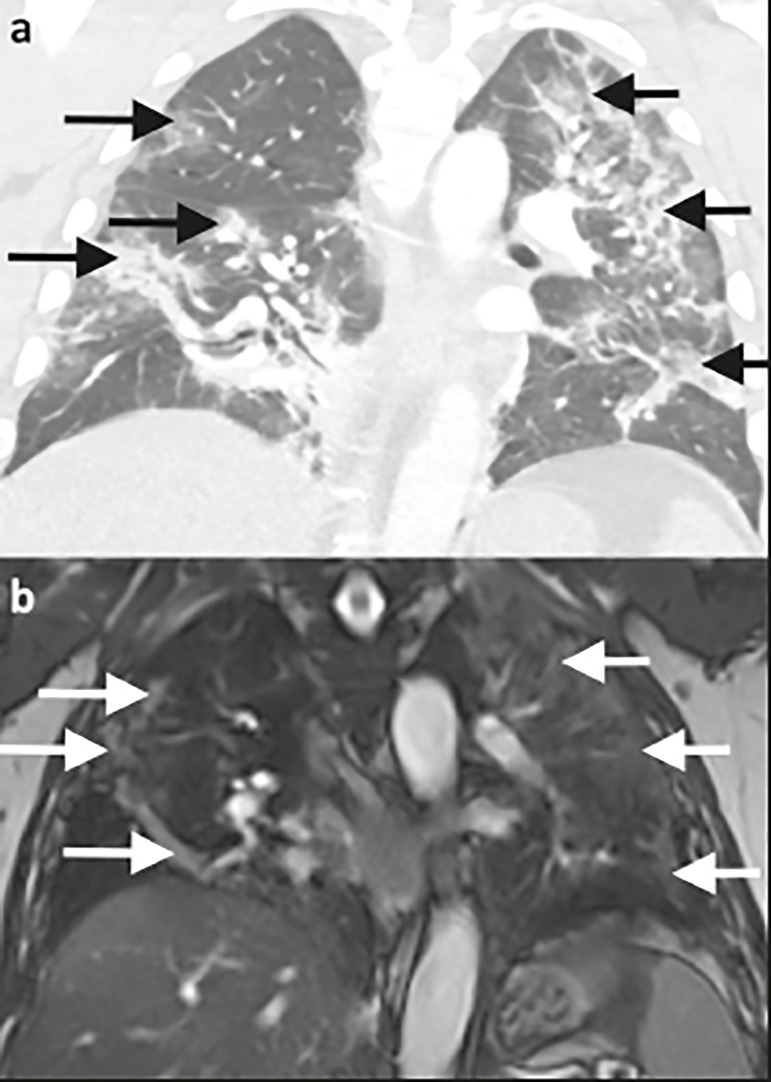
Ability of 1.5 T TrueFISP MRI to show the distribution of pulmonary findings. CT and MRI scans (a and b, respectively) showing patchy and linear consolidations and ground-glass opacities in the upper, middle, and lower lobes, as well as in the central and peripheral zones of the lungs (arrows). The CT scan was acquired on the day of COVID-19 confirmation by RT-PCR. MRI was performed 17 days after the CT.
